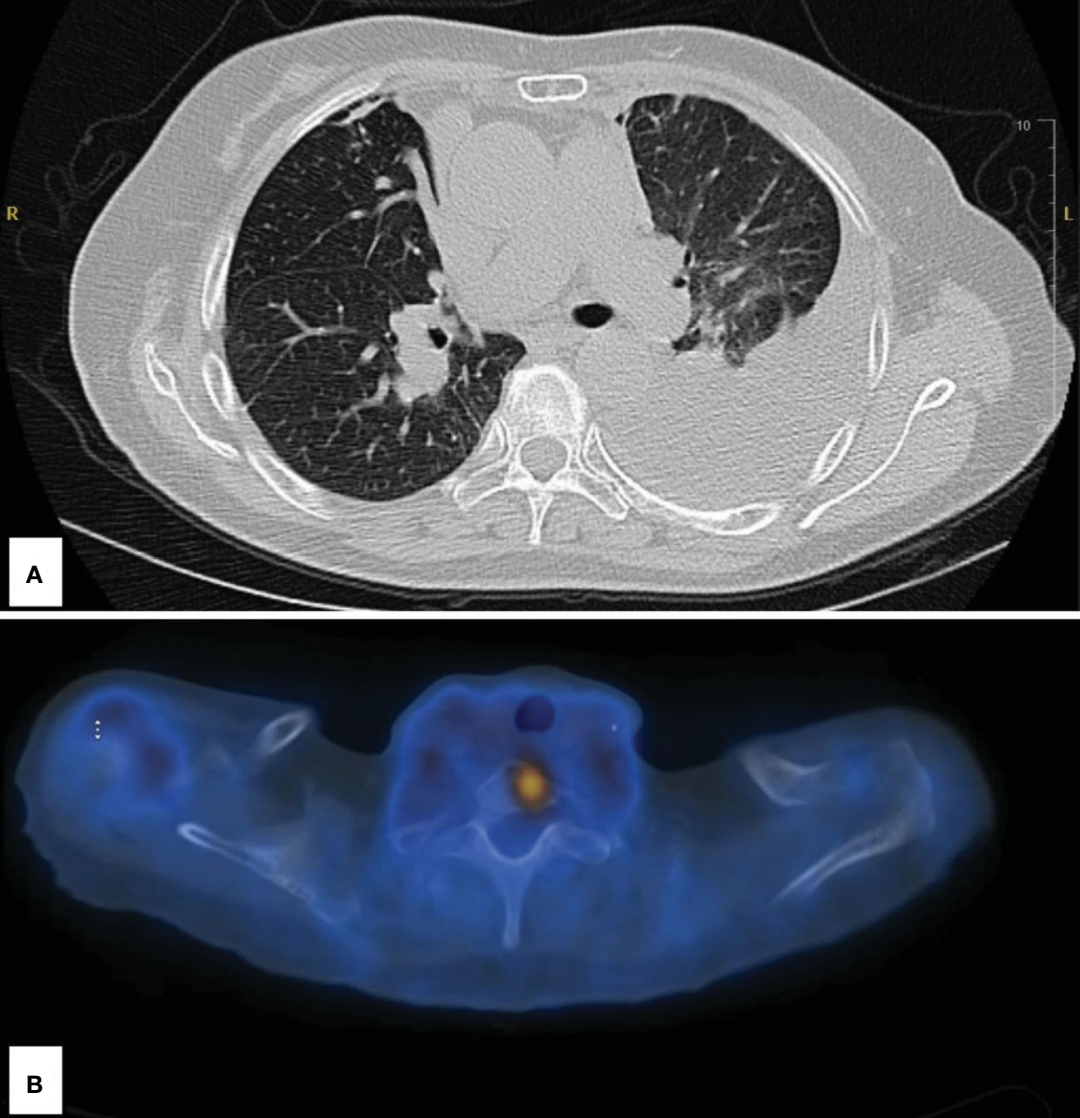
Imaging following disease progression at 2 years’ status post-stereotactic body radiation therapy. (A) Large left pleural effusion and left lower lobe atelectasis. (B) Abnormal fluorodeoxyglucose uptake in the upper spine; SUV of 5.5.
